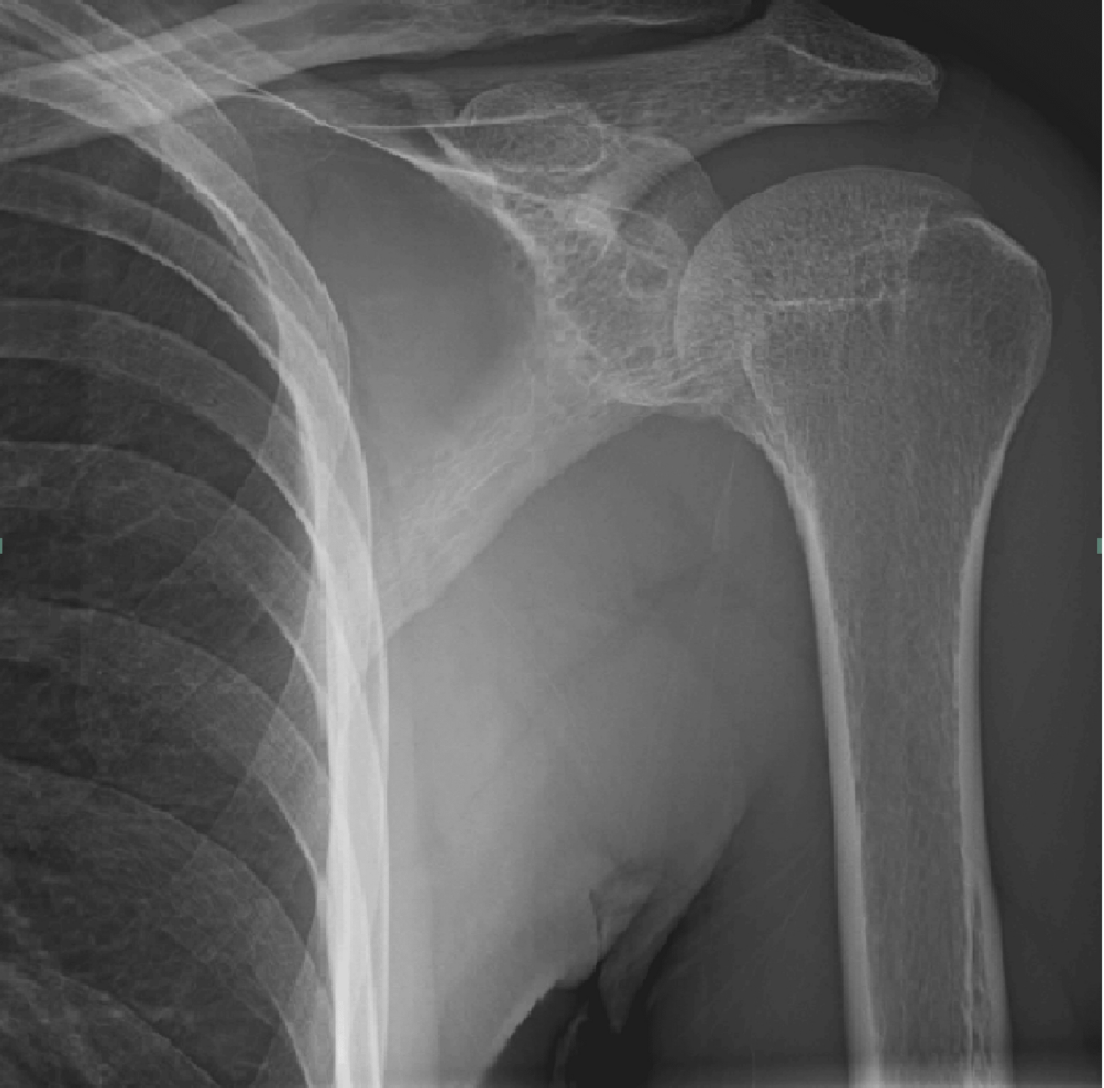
Repeat left shoulder X-ray The acromioclavicular and glenohumeral joints remain in proper anatomic alignment
